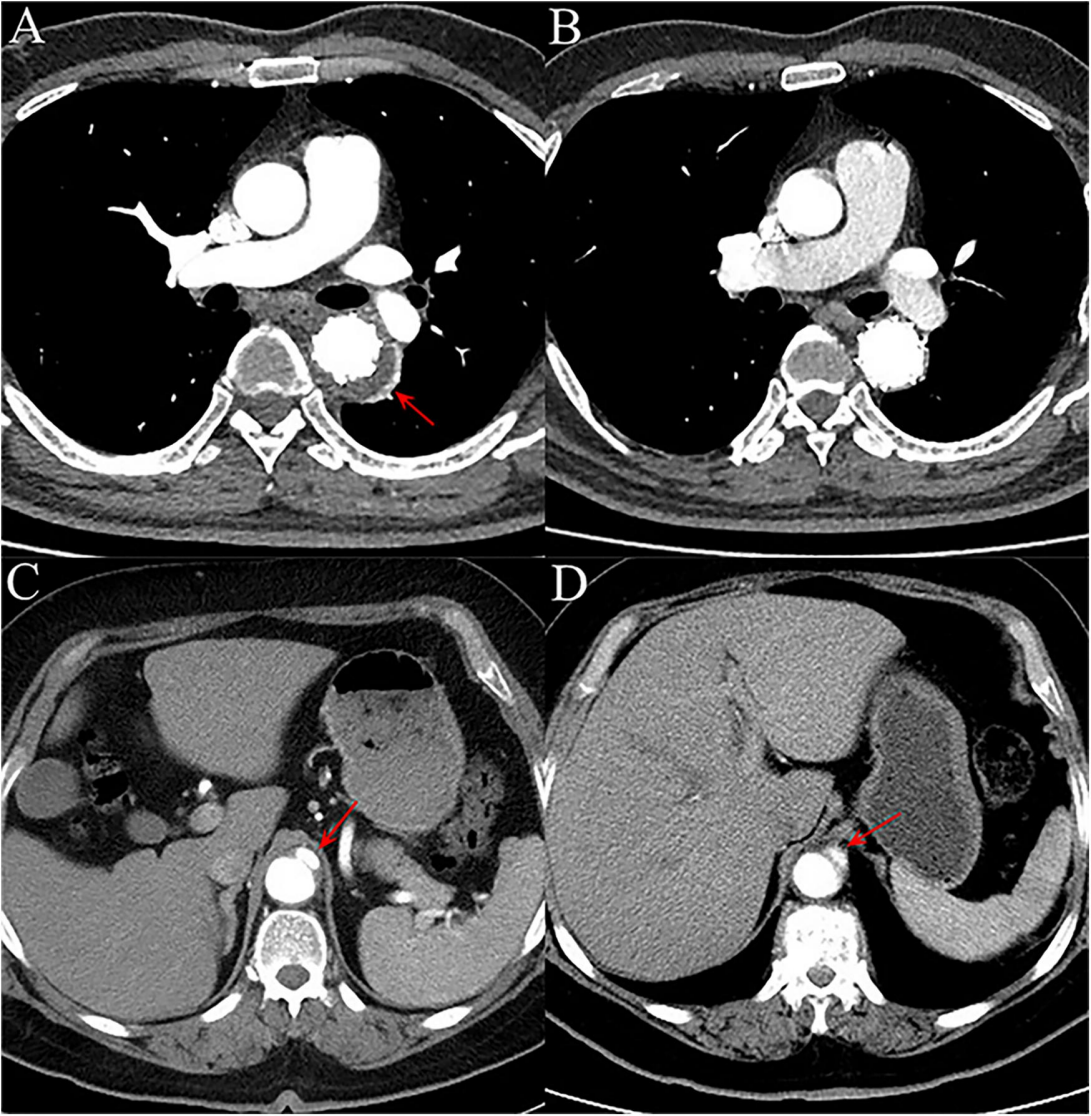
(A) on aortic computed tomography angiography (CTA) performed within 30 days after the procedure, a type Ib endoleak (red arrow) was observed in one patient, with contrast agent leaking into the aortic mid-layer from the distal landing zone of the endograft. (B) The endoleak had disappeared by the 1-year follow-up CTA. (C) In another patient, CTA within 30 days post-operation revealed a new-onset focal aortic dissection (red arrow) originating from the distal landing zone of the endograft, without involvement of major branch vessels. (D) At the 1-year follow-up, the aortic dissection (red arrow) remained stable in extent, with no significant progression.
